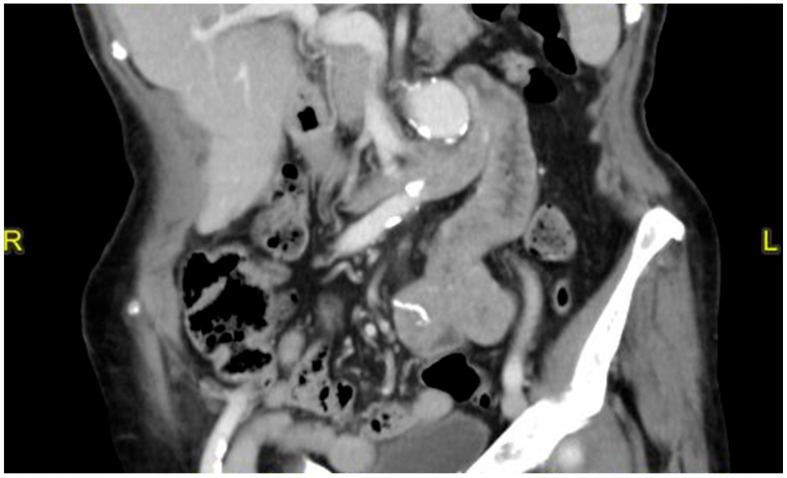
Angio CT showing multiple hyperdense endoluminal images at the fourh duodenum and first jejunal loop up to surgical anastomosis referable to contrast spillage.
